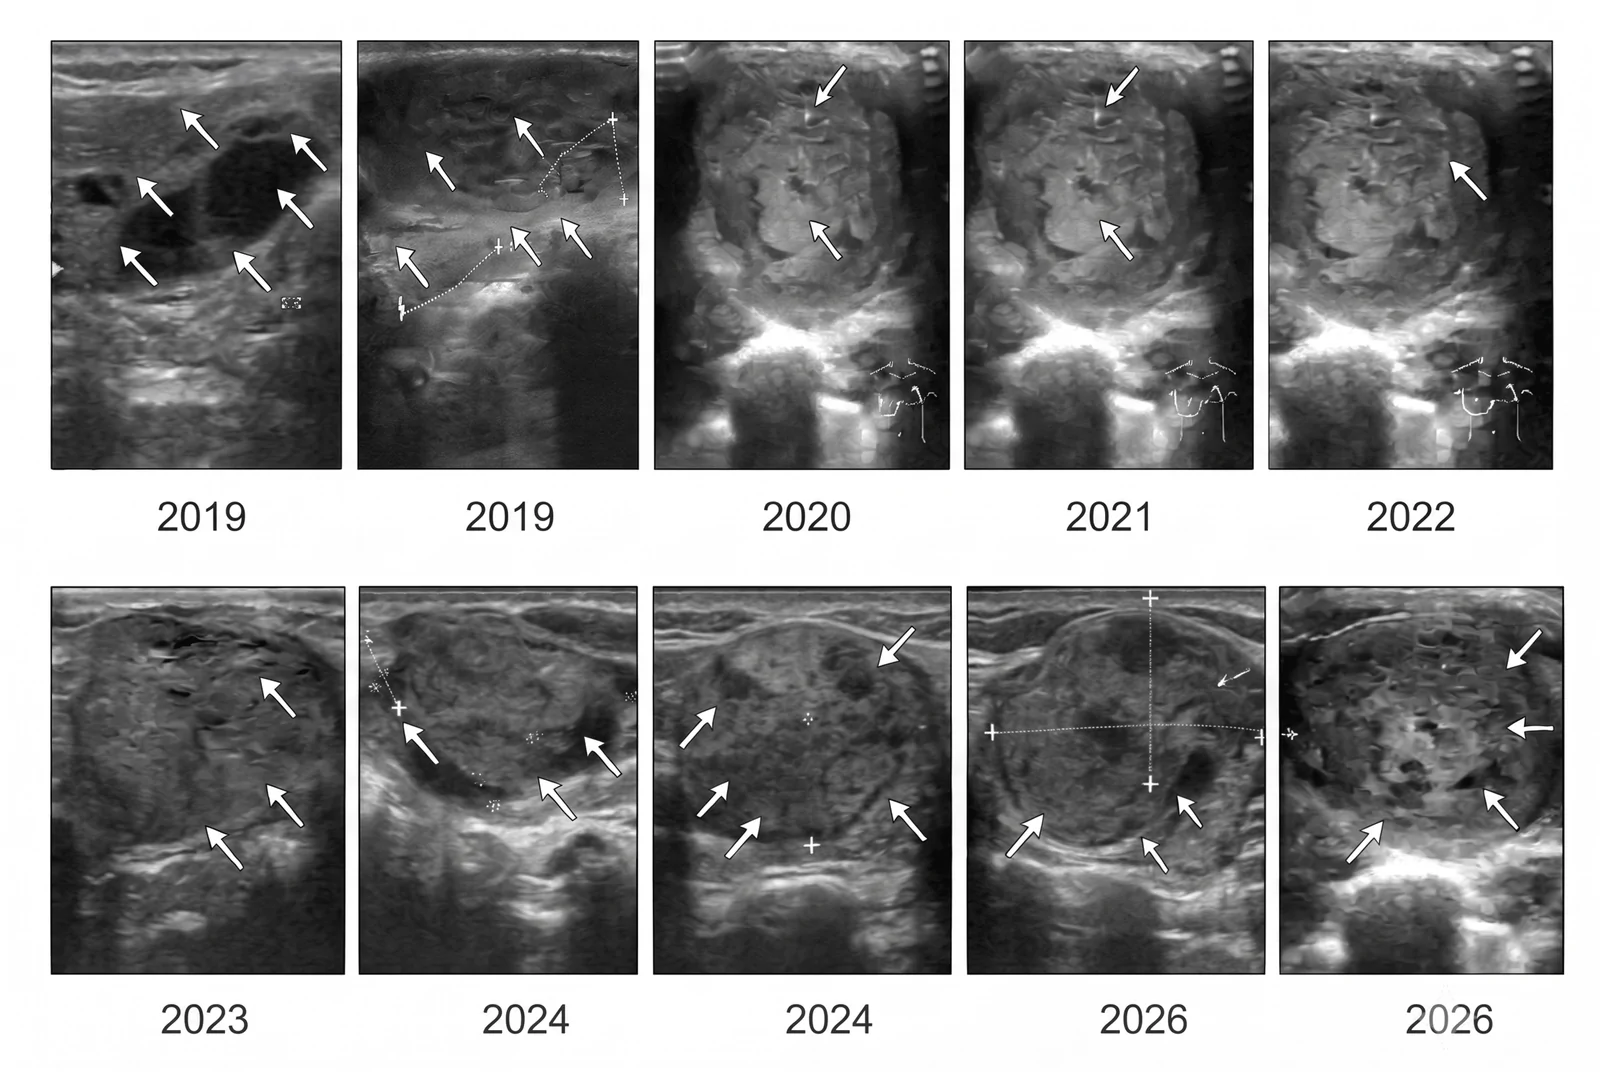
Serial USG images demonstrating the longitudinal progression and morphological changes of the left axillary/chest wall lesion from 2019 to 2026. Chronological USG series spanning seven years (2019-2026) detailing the gradual enlargement and structural evolution of the left axillary/chest wall mass. The arrows indicate the boundaries and internal features of the lesion, showing progressive changes from initial fluid-debris components and thick-walled collections to a larger heterogeneous mass with increased solid components. USG, ultrasound
